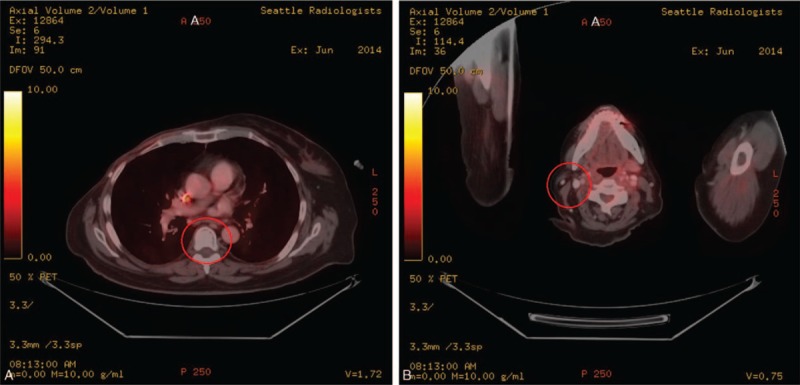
Positron emission tomography and computed tomography axial fused images from June 2014 showed interval sclerosis of the lesion within the T6 vertebra without focal radiotracer accumulation within this bone lesion. There was some focal radiotracer accumulation within the distal tip of the left portacatheter within the superior vena cava which was noted to be due to a small thrombus (A). The previous metabolically active right level IIB lymphadenopathy had resolved (B).
